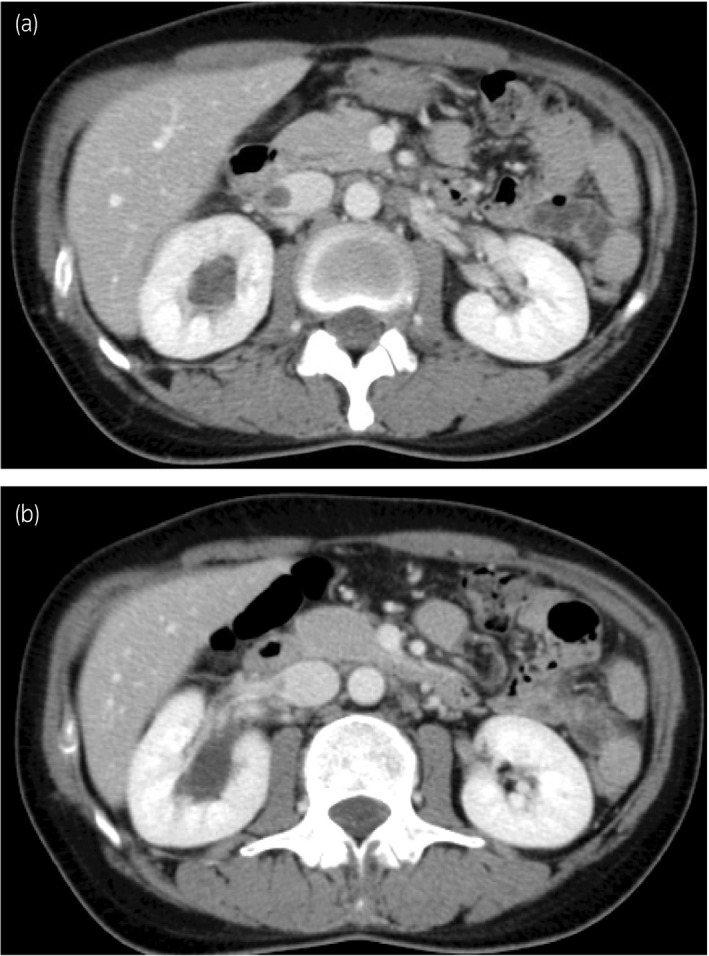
Axial images during the nephrographic phase of contrast‐enhanced CT before ureteroneocystostomy. (a) Mild, right hydronephrosis with homogeneous renal parenchymal enhancement and the low‐density lesion in the IVC, wherein densities in both lesions are very similar. (b) The low‐density lesion in the IVC and dilated renal pelvis appears to be continuous through the renal vein.
